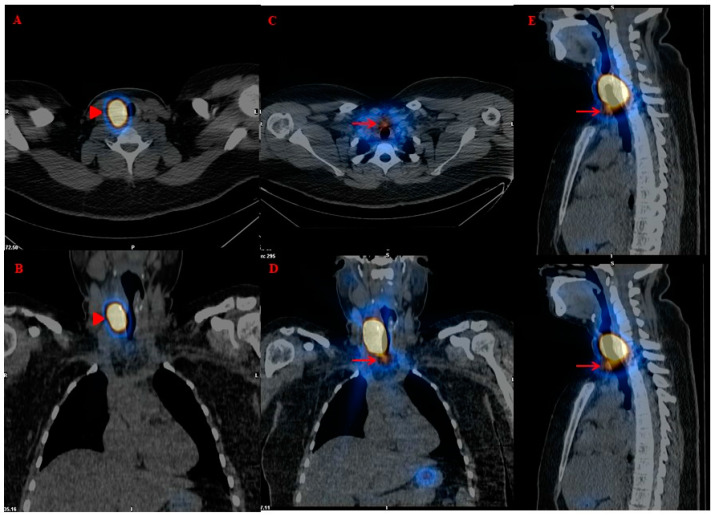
A twenty-six-year-old woman affected by multifocal papillary thyroid carcinoma (12 mm in maximum size), follicular variant, located in the lower part of the left lobe (pT1b(m), Nx, Mx). Postoperative neck-ultrasonography (nUS) performed within three months after thyroid surgery did not show any thyroid remnant and/or suspicious or pathological loco-regional lymph node. The patient underwent rhTSH-aided (standard protocol) iodine-131 therapy (RIT) with ablative purpose (2.2 GBq). At RIT, the peaks of TSH 65 μIU/mL, TgAb 13.6 IU/mL, and basal and stimulated Tg values (day 1, 3, and 5) were <0.04, 3.2, and 10.3 ng/mL, respectively. (A,B) pT-WBS (anterior and posterior views) and static images (anterior and posterior views) of the neck–thoracic region were obtained two days after RIT. An intense radioiodine uptake consistent with thyroid remnant parenchyma was noted in the right thyroid bed ((A,B) arrowheads). In addition, a small-sized radioiodine avid lymph node metastasis (red arrows) located in the pre-tracheal region (i.e., VI Robbins’ level) was noted (C–E).
